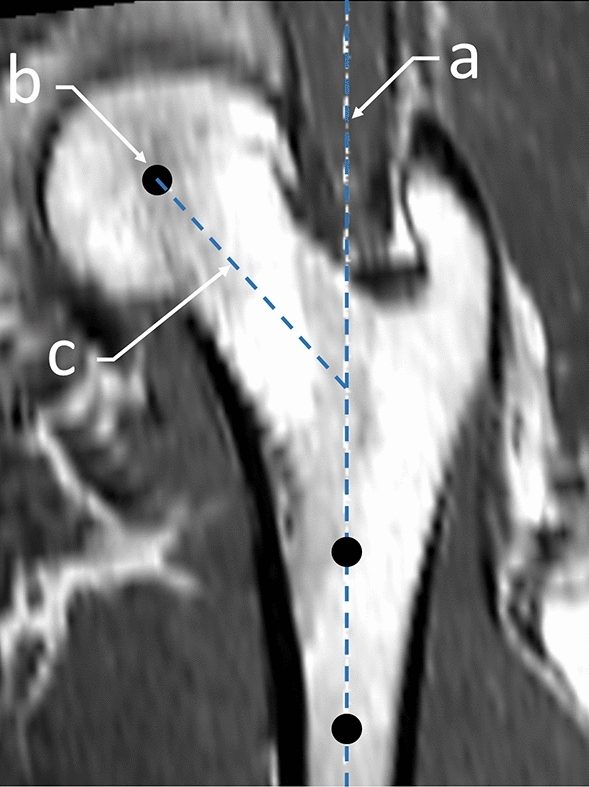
MRI scans were aligned into a common 0° orientation (shown) and then rotated into fall and stance configurations prior to FE model generation. Using the segmented mask data, the long axis of the femur (aka shaft axis) (a) was defined by identifying the line-of-best-fit through centroids of axial slices distal to the greater trochanter. The center of the femoral head (b) was identified by fitting a sphere to the surface of the head via a variant of the iterative closest point algorithm. A vector corresponding with the neck was also defined by identifying the line-of-best-fit through centroids of slices in an axial-oblique orientation. This vector was then projected to a plane containing the shaft axis and center of the femoral head. The neck axis (c) was defined as the projected vector passing through the femoral head and intersecting with the shaft axis. This configuration was used to define the common 0° orientation with the shaft axis aligned vertically and the neck axis aligned with 0° internal/external rotation.
